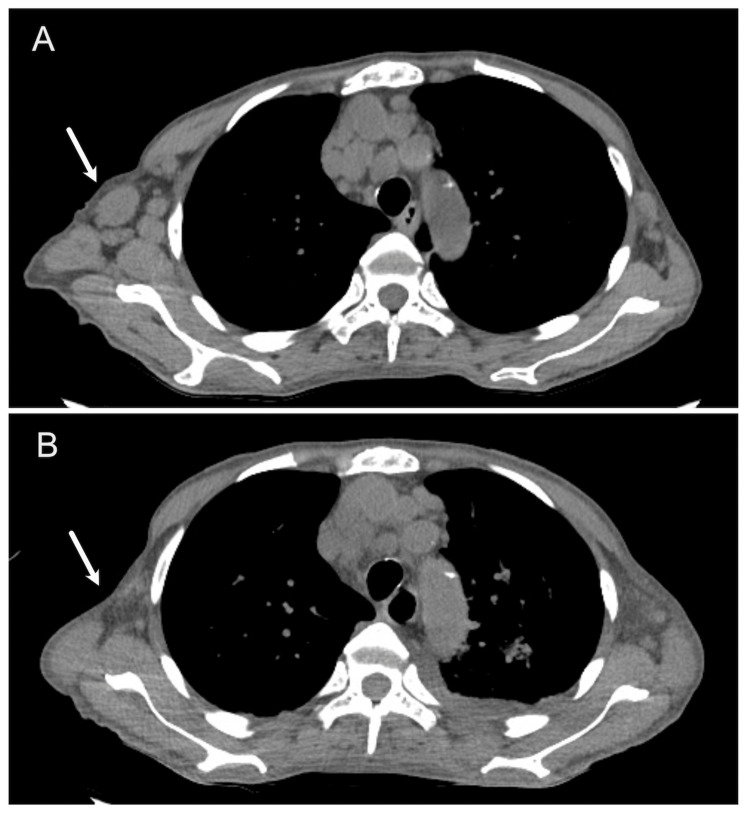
Results of CT scans (A) CT scans revealed systemic lymphadenopathy (arrow: axillary lymph nodes) at the time of admission. (B) CT scans after dexamethasone induction exhibited a mild reduction in the size of multiple lymph nodes.
